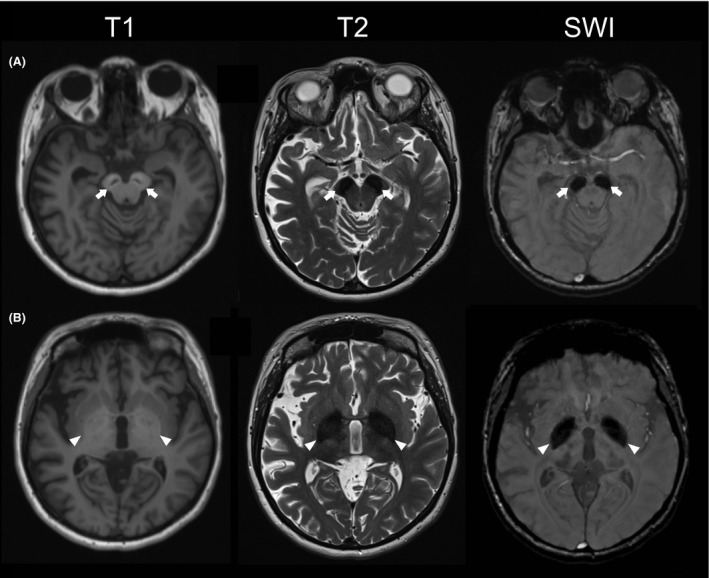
MRI findings in BPAN. Brain MRI of the Norwegian patient at the age of 33 years, showing typical findings for BPAN. (A) Axial T1‐weighted image at the level of the midbrain shows symmetric hyperintense “halos” surrounding a band of central hypointensity in the *substantia nigra* (arrows). Axial T2‐weighted and SWI image of the same area shows prominent hypointensities in the *substantia nigra* and cerebral peduncles (arrows). (B) Axial images at the level of the striatum show high T1 signal and low T2 and SWI signal in the *globus pallidus*. The low SWI signal corresponds to areas of increased iron deposition (arrowheads).
